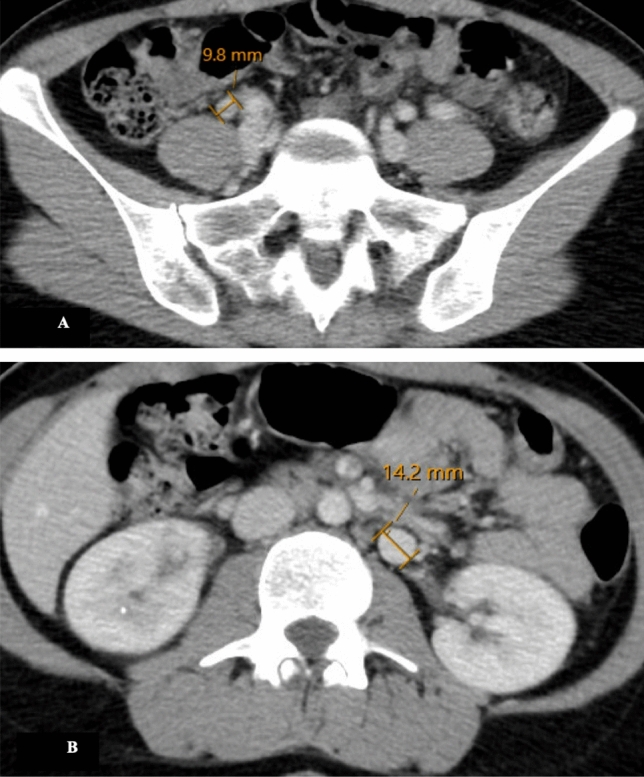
Dilated gonadal veins in asymptomatic patient. 22-year-old nulliparous female presenting with 3–4 days of intermittent right-sided abdominal pain and no prior symptoms of chronic pelvic pain. She was found to have pyelonephritis with incidental findings of bilateral gonadal vein dilation. Axial contrast-enhanced computed tomography with measurement calipers (orange) shows a right gonadal vein measuring 9.8 mm (A) and left ovarian vein measuring 14.2 mm (B)
